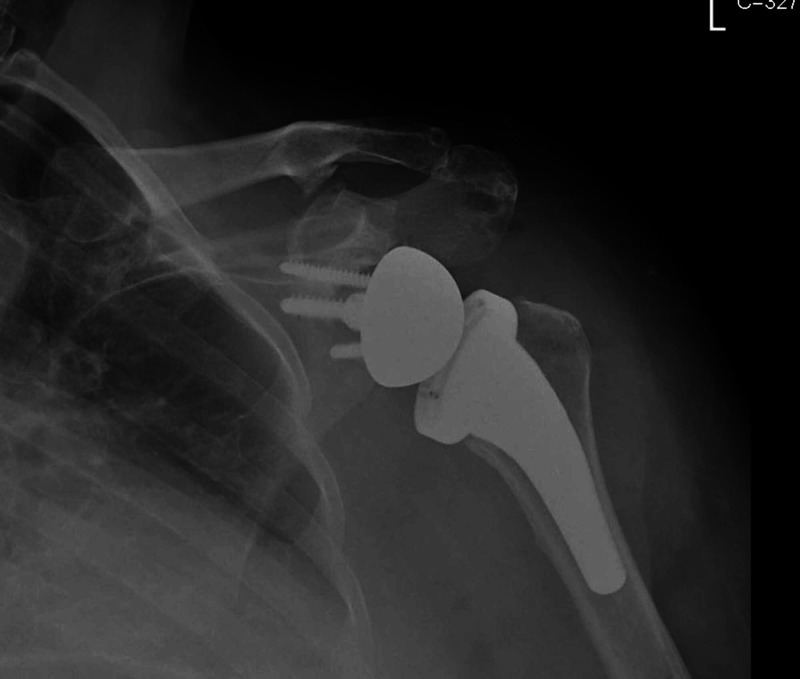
Initial post-operative plain radiograph of the left shoulder (AP view) AP: anteroposterior
